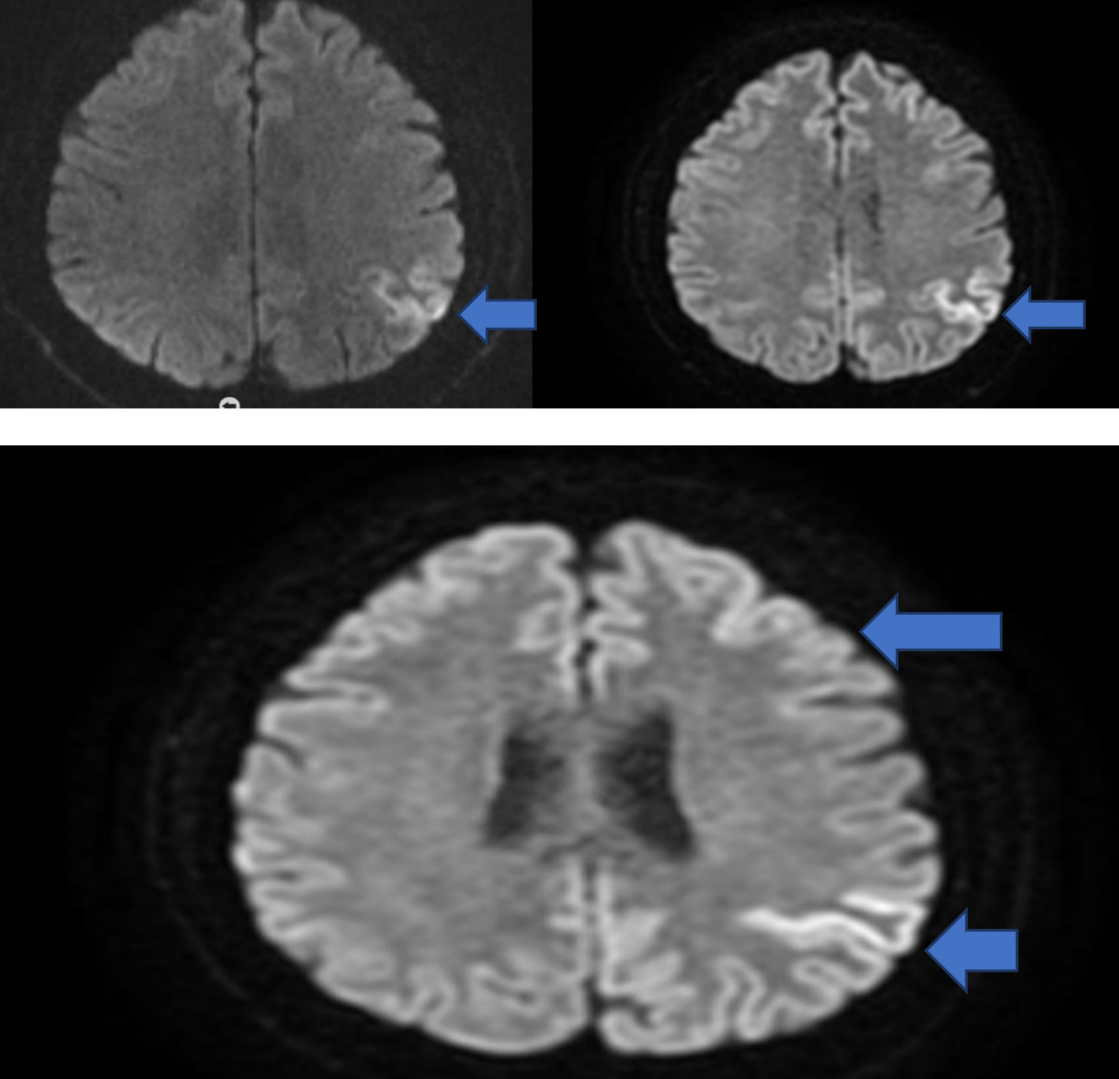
Cortical diffusion restriction in the left parietal region in brain MRI diffusion-weighted imaging.
